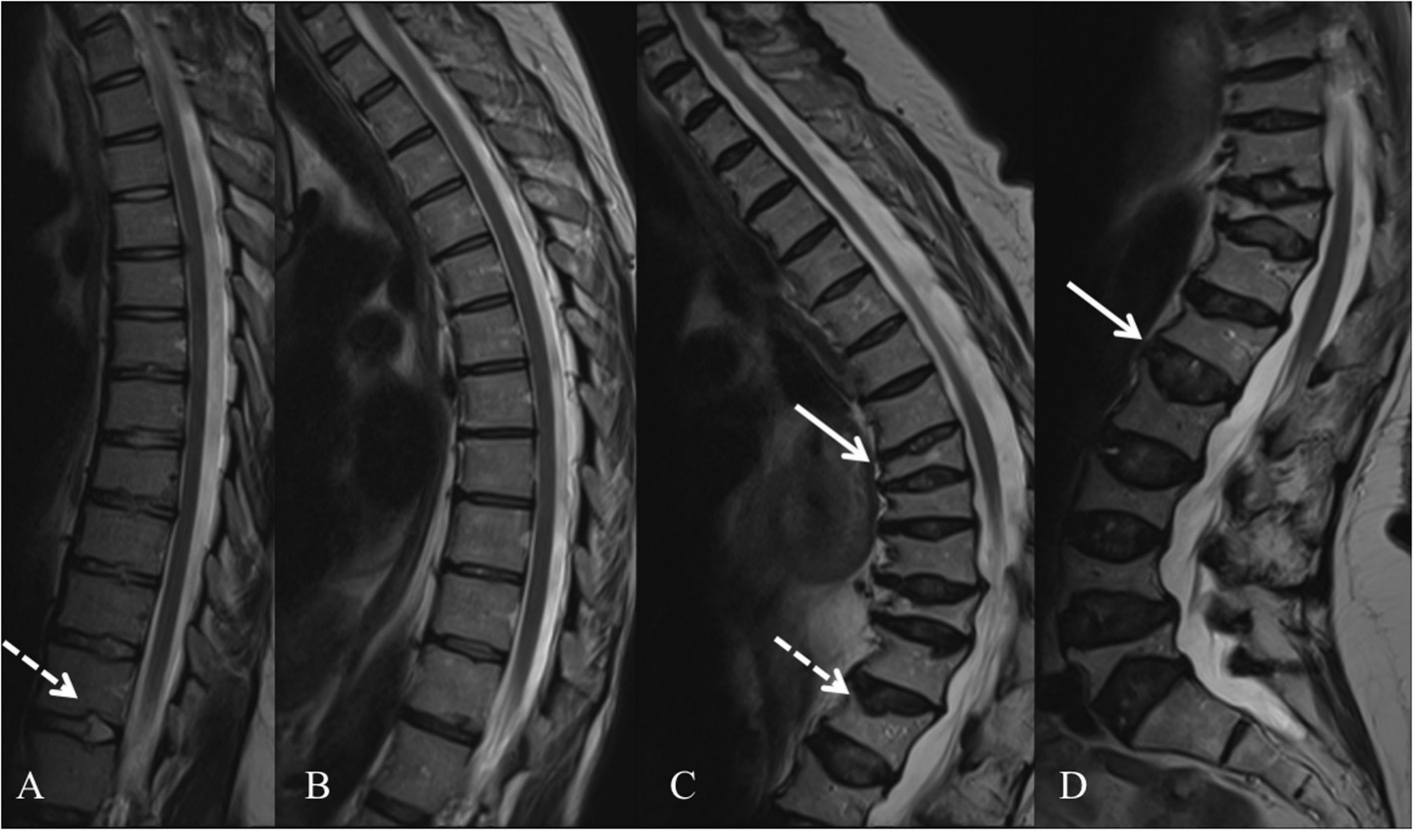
Spinal magnetic resonance images of four *WNT1* p.C218G mutation-positive subjects. (A) Thoracic spine of a 17-years-old female showing multiple Schmorl nodes (arrow). (B) Thoracic spine of a 44-years-old female showing exaggerated thoracic kyphosis. (C) Thoracic spine of a 76-years-old male showing several compressed vertebrae, kyphotic stature, and Schmorl hernia (arrow). (D) Lumbar spine of a 74-years-old female showing several compressed vertebrae and enlarged intervertebral discs (arrows). Reprinted from Mäkitie et al. (63) with permission from Elsevier.
